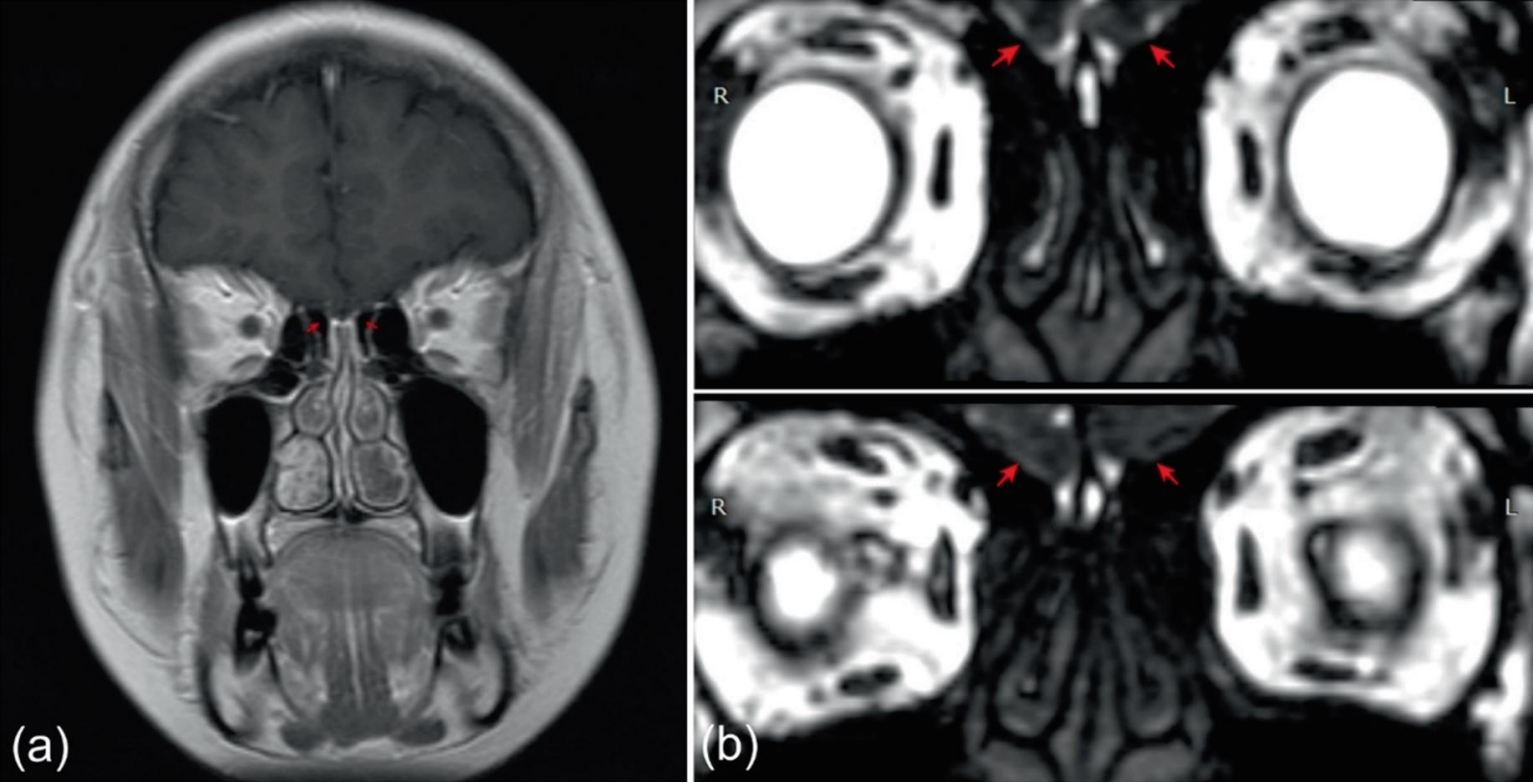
Magnetic resonance imaging (MRI) of the anterior cranial fossa. (a) T1 coronal sequence and (b) CISS sequence. Red arrows indicate the absence of the olfactory bulbs, consistent with olfactory bulb aplasia. The olfactory sulci were only partially captured and could not be reliably evaluated.
